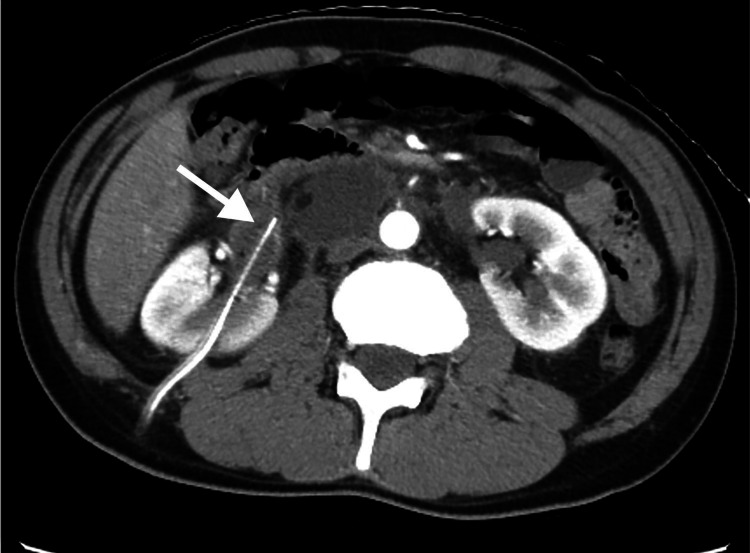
Abdominal CT on day 7 demonstrates a percutaneous nephrostomy catheter placed in the right renal pelvis (arrow).
